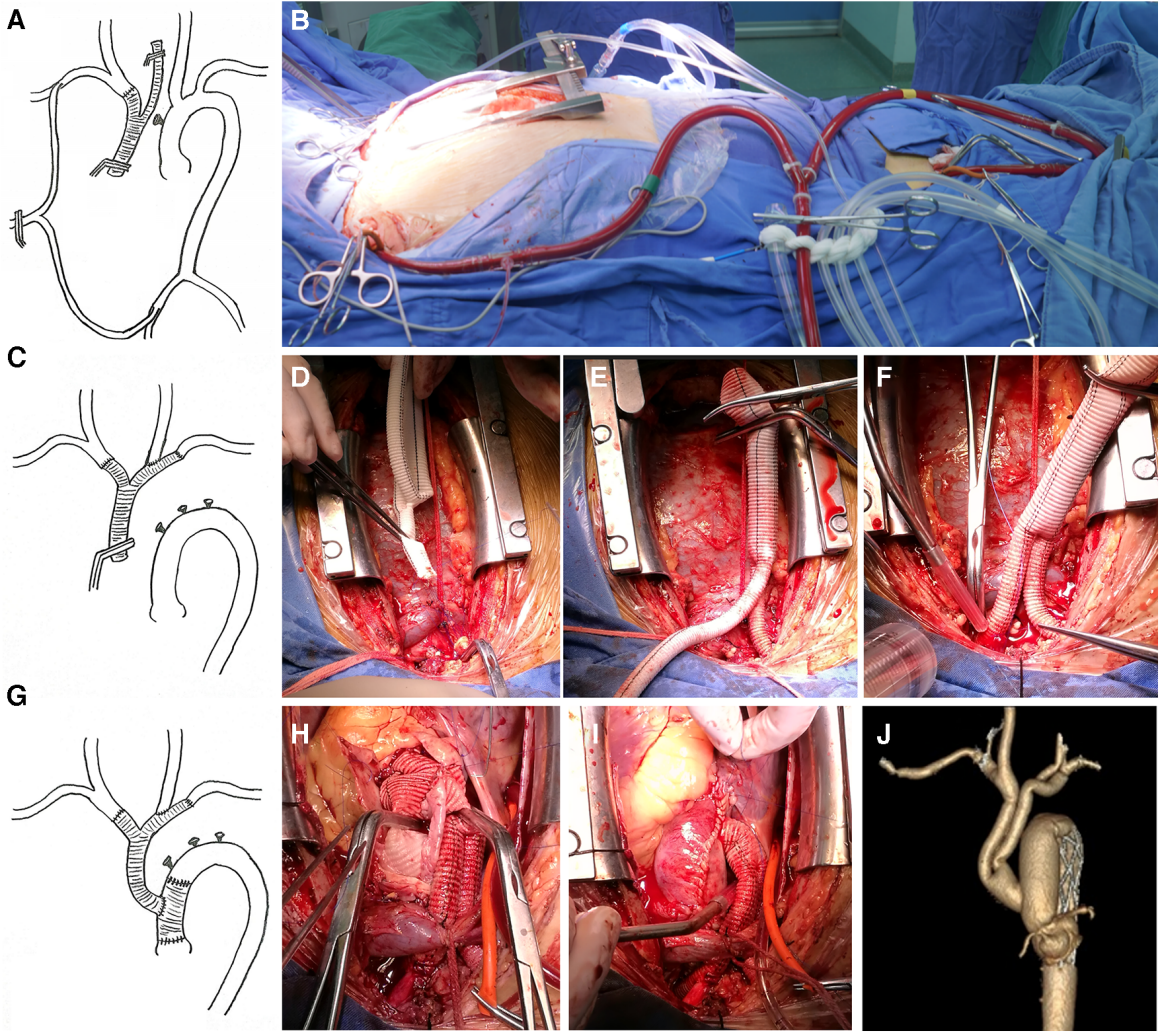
(A,B) The right axillary artery and the right common femoral artery are cannulated separately, and the two cannulas are connected through an arterial perfusion catheter of the CPB. The bypass from the right femoral artery to the axillary artery is thus established, (D–F). The UA, LSCA, and LCCA are sequentially reconstructed by supplying blood to the brain through the bypass, (G–I). After completing the replacement surgery of the ascending aorta, the Y-shaped graft is anastomosed end-to-side with the ascending aortic graft, (J). Postoperative CTA imaging.
